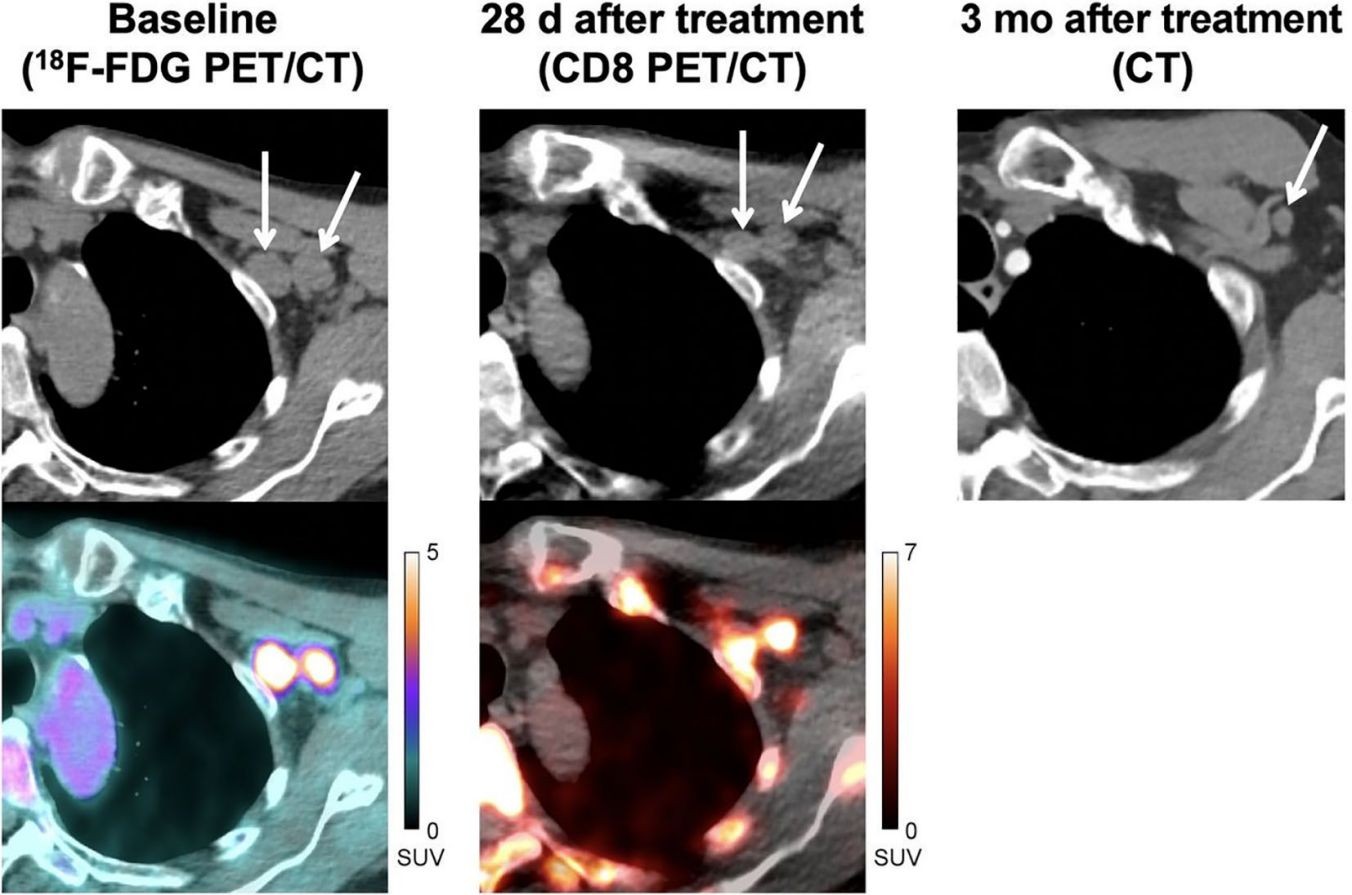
A 71 years old patient (locally advanced stage III melanoma) with immunotherapy (pembrolizumab). CD8 PET/CT images, which performed 28 days after pembrolizumab, show evident uptake in metastases indicating significant infiltration of CD8^+^ T cells. CT images performed 3 months after treatment confirmed the efficacy of immunotherapy. Reproduced from Farwell MD et al. (97).
